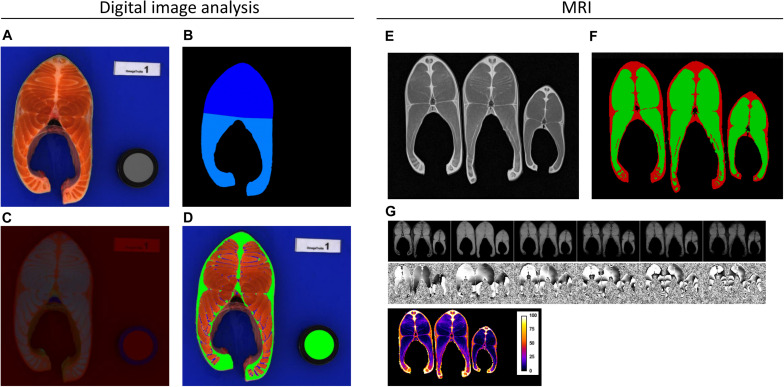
Examples of images obtained using digital image analysis (A) Steak prior to image analysis. (B) Dorsal and ventral segmentation. (C) Image for L*a*b analysis. (D) Differentiation among adipose tissue (green), myosepta (blue), and flesh (red). using MRI (E) ‘Spin echo’ MRI image. (F) Differentiation between subcutaneous fat (red) and flesh (green). (G) Module and phase images, and map of lipid percentage in a whole steak.
